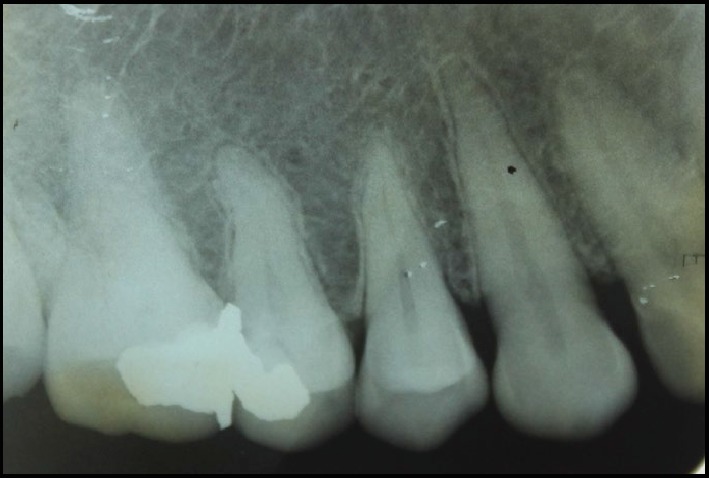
IOPA radiograph of 13–15 region.
